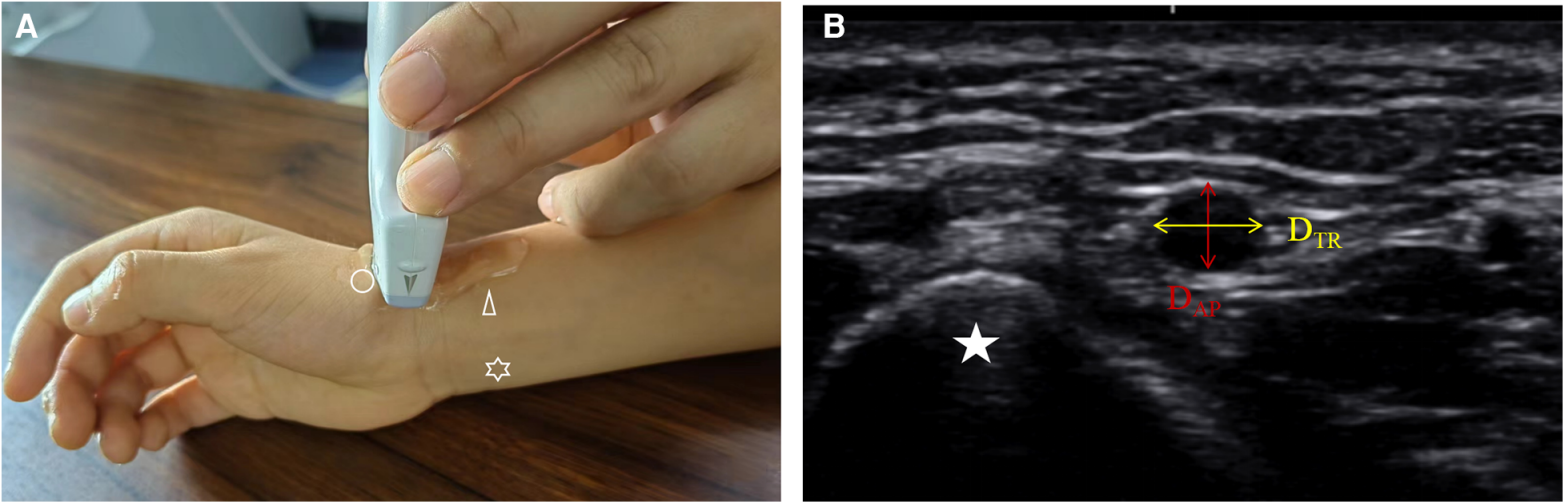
The measurement of DRA diameter in children by ultrasound. (A) the site of ultrasound measure. Circle: distal radial artery; Triangle: proximal radial artery; Star: ulnar artery. (B) the cross-sectional image of the distal radial artery in anatomic snuffbox. Star, scaphoid; D_TR_, transverse diameter; D_AP_, anteroposterior diameter.
